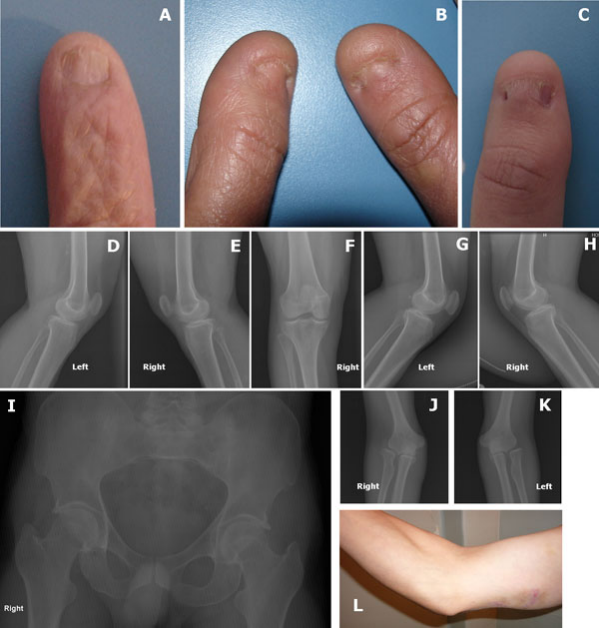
Photographs of the nails and radiographs of knees, hips and elbows of some of the subjects. Nail involvement in these cases includes dystrophic, thin, hypoplastic discolored nails with longitudinal ridges. Case III-2 shows decreased creases over the skin of distal interphalangeal joints, which is a sensitive sign of digital involvement in this patient (A). Thumbs are most severly affected in case III-7 (B), and IV-9 (C). The radiographs of knee involvement in Case III-2 shows bilateral involvement of the patella, with hypoplastic, higher than normal missplaced patella (D-F). A small 6 mm bone fragment can be observed on the lateral border of right patella (E). Knee radiographs of Case III-7 show slight bilateral patellar hypoplasia, mostly on the transverse diameter (G and H). Hip radiography of Case IV-2 shows loss of the normal concavity at the junction between the head and femoral neck bilaterally. The radiographs reveal an elbow involvement of subject IV-2 (J and K) and include a prominent medial epicondyle and hypoplasia of the capitellum on both sides (J and K). They also show a hypoplasia of the lateral epicondyle and capitellum with a slight deformity of the radial head (J and K). Underdeveloped triceps and prominent medial epicondyle are observed (L).
